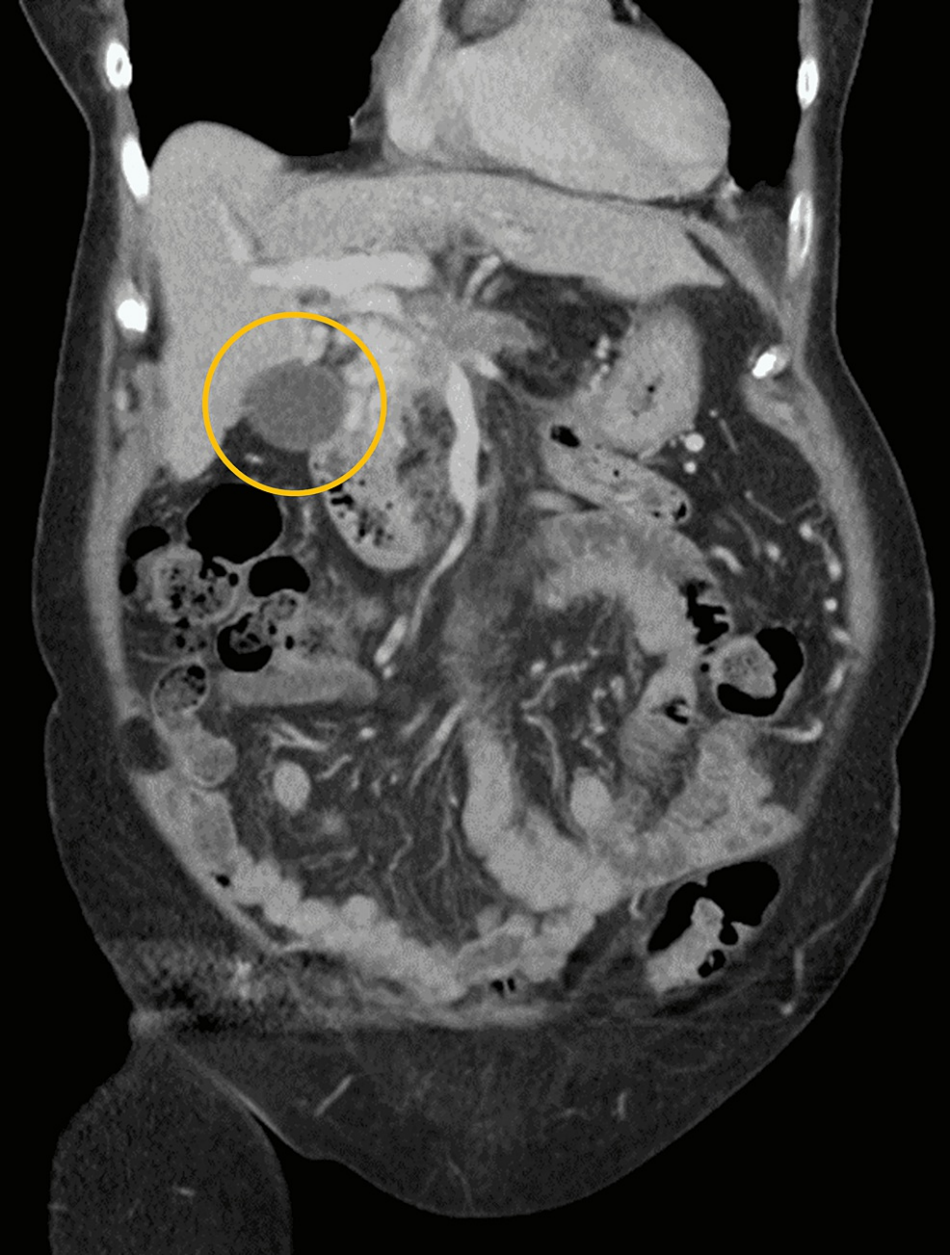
CT scan showing pancreatic head mass highlighted by the yellow circle
